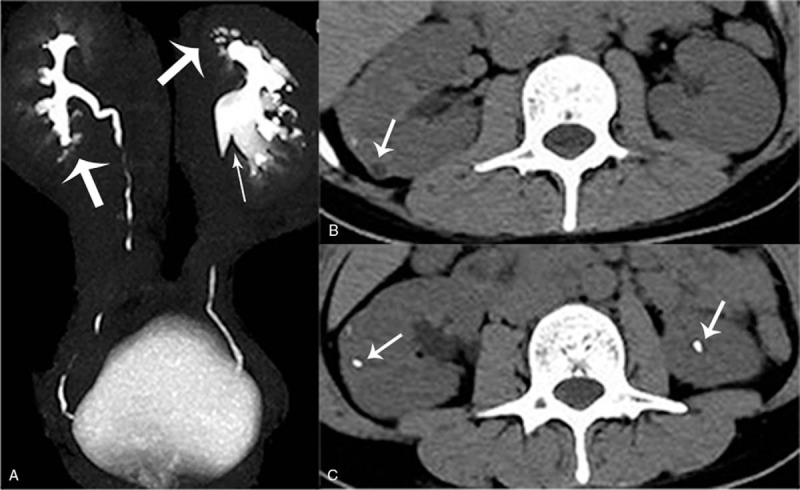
Computed tomography urography (CTU). (A) Dilated pelvis and calyces of right renal (thin arrow); characteristic imaging of dilated collecting tubules in bilateral kidneys with a “papillary brush” appearance (thick arrows); (B) Medullary cyst (arrow); (C) Enlarged right renal and multiple nephrolithiasis (arrows) in the bilateral renal parenchyma.
